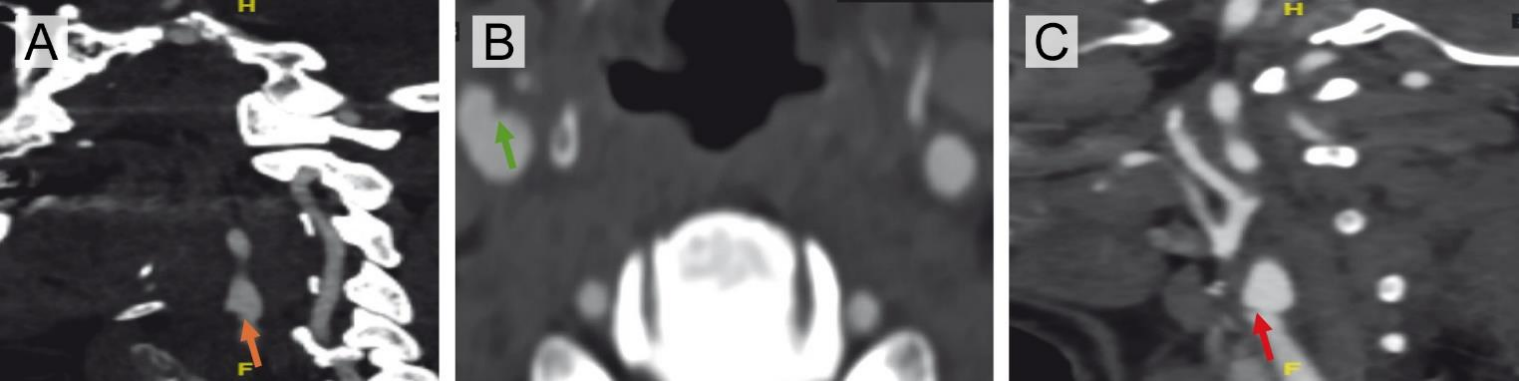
Figure 3. Interval development of thrombus over a carotid artery web in a patient with recurrent ischemic events. (A) Axial CTA at the time of large vessel occlusion demonstrates a carotid web in the right internal carotid artery with superimposed thrombus (orange arrow). (B) Corresponding sagittal view confirms the presence of thrombus overlying the web in the proximal right internal carotid artery (green arrow). (C) Imaging performed three weeks earlier during workup for TIA shows the same carotid web without thrombus (red arrow).
